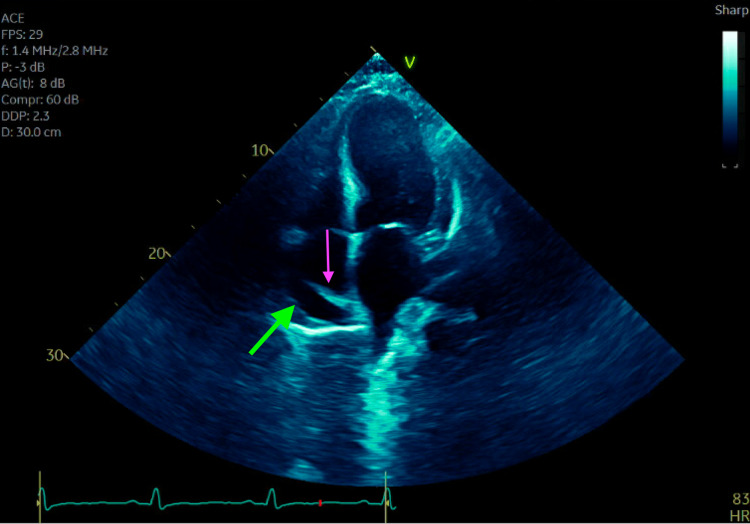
Trans-thoracic Echocardiogram (TTE) View of Pericardial Effusion Still image of the apical four-chamber view of the patient's transthoracic echocardiogram demonstrating a small pericardial effusion (green arrow) and diastolic collapse of the right atrium (pink arrow).
